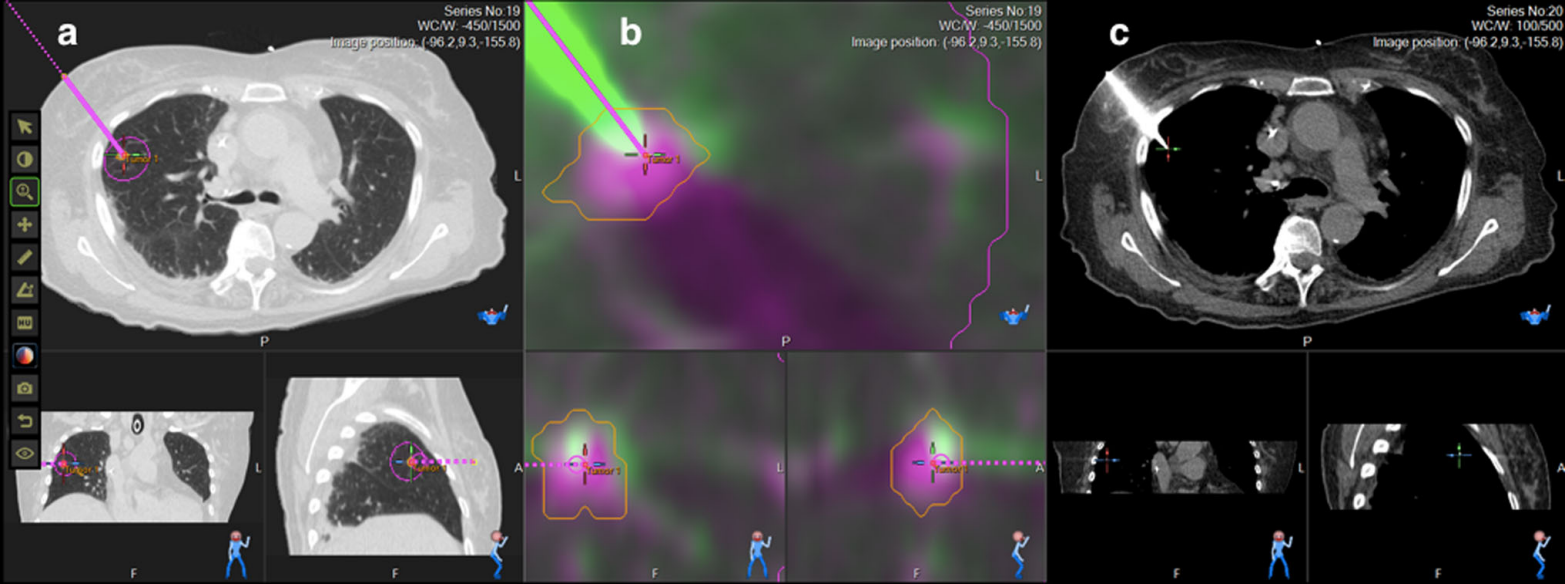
Example images from the robotic workstation, during a radiofrequency lung ablation in a 76-year-old woman with a 6 mm rectal cancer lung metastasis in the right upper lobe. (a) unenhanced planning CT with multiplanar reformats, showing the planned needle path in magenta (b) fused planning and needle placement CTs with multiplanar reformats, showing the planned needle path in magenta, and actual needle placement in solid green (c) multiplanar reformats of needle placement CT
